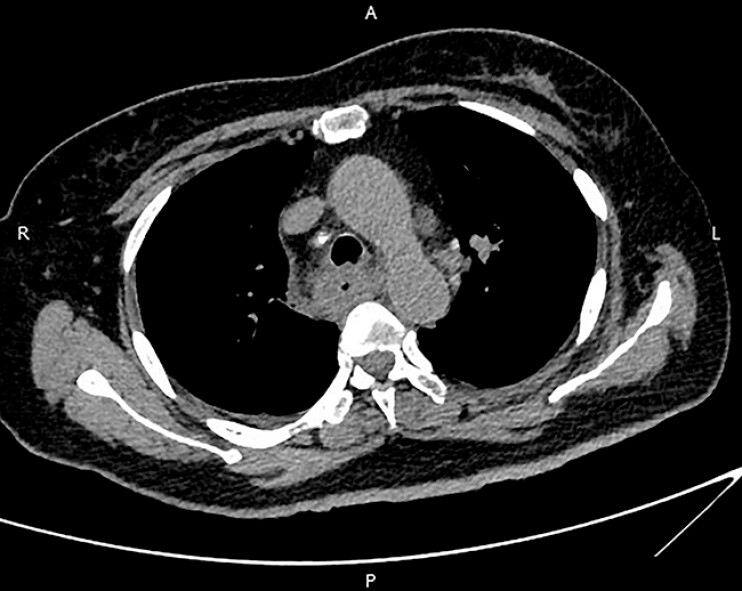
Computed tomography of the chest showed a thickened wall of the entire esophagus and blurring of the peri-esophageal fat space.
